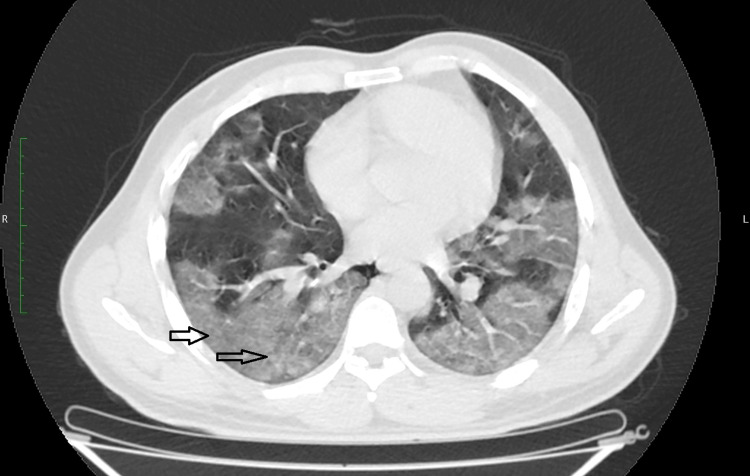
CT Chest Showing Heavy Opaque Consolidation and Marked Septal Thickening
